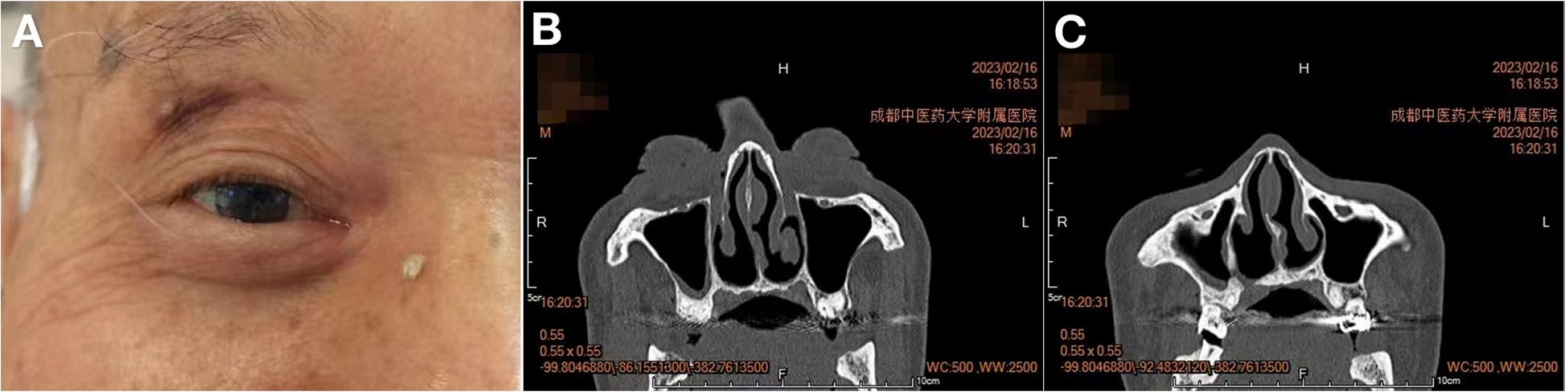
The patient's eye appearance and sinus CT images at 1 month after surgery. A The patient showed no symptoms of lacrimation or purulence in the right eye. B Horizontal CT images showed that the right sinus and nasolacrimal duct had a uniform density and that the nasolacrimal duct was patent. C Horizontal CT images showed no high-density or soft-tissue-density shadows in the right nasal cavity
